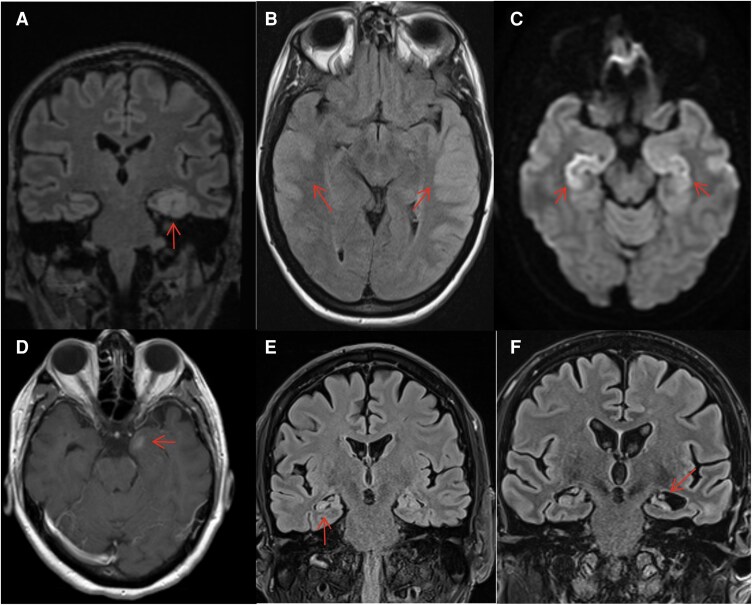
Representative MRI images with relevant abnormalities. Abnormalities are indicated by arrows, and demonstrate: (A) left sided hippocampal swelling and FLAIR hyperintensity in a patient with anti-LGI1 Ab-mediated encephalitis; (B) bilateral temporal FLAIR hyperintensity in a patient with anti-LGI1 Ab-mediated encephalitis; (C) bilateral hippocampal diffusion restriction in a patient with anti-LGI1 Ab-mediated encephalitis (associated with ADC reduced signal bilaterally); (D) left amygdala T1-weighted contrast enhancement in a patient with anti-AMPAR Ab-mediated encephalitis; (E) right sided hippocampal atrophy without sclerosis in a patient with anti-LGI1 Ab-mediated encephalitis; (F) left hippocampal sclerosis in a patient with anti-LGI1 Ab-mediated encephalitis. Ab, antibody; ADC, apparent diffusion coefficient; AMPAR, α-amino-3-hydroxy-5-methyl-4-isoxazolepropinoic receptor; FLAIR, fluid-attenuated inversion recovery; LGI1, leucine-rich glioma-inactivated 1.
